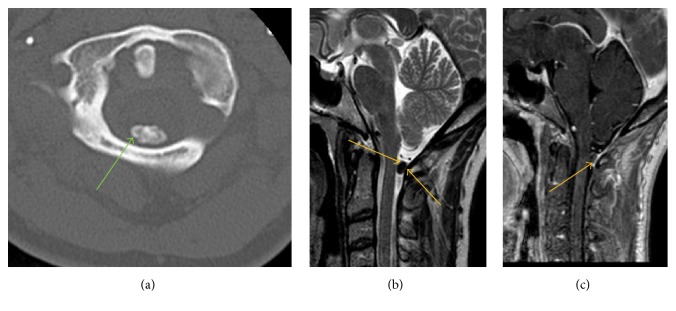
Axial NECT of cervical spine (Figure 6(a)) demonstrates a calcified well-marginated lesion (green arrow) within posterior aspect of central canal at C1 level. On sagittal T2WI (Figure 6(b)) and sagittal postgadolinium (Figure 6(c)) MR images, this extramedullary intradural lesion (orange arrows) is noted to be T2 hypointense and mildly rim enhancing, respectively, causing effacement of posterior thecal sac and abutment of cervical spinal cord at the level of posterior arch of C1. The imaging findings favor a calcified meningioma.
